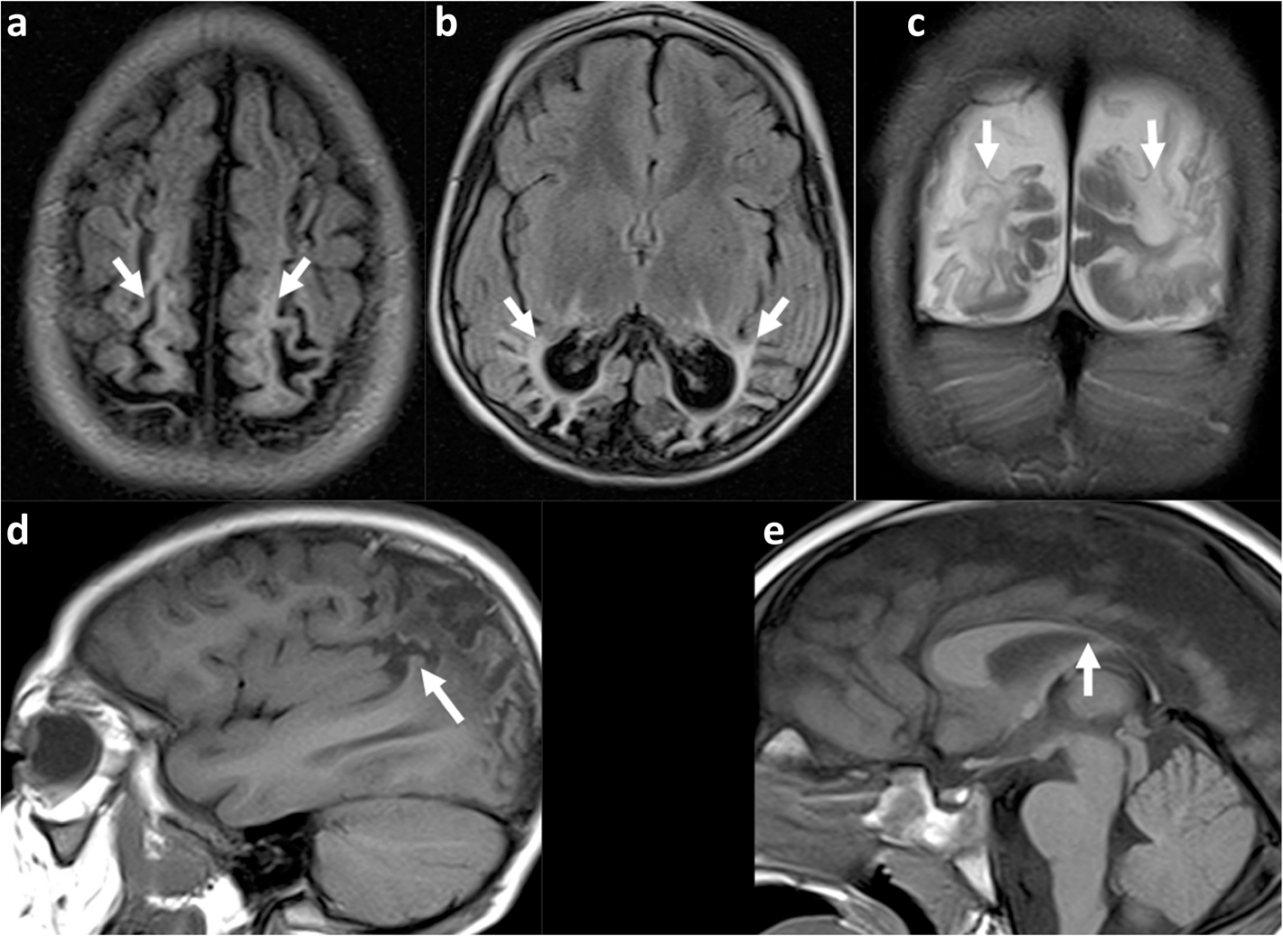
a–e Peri-Sylvian and posterior watershed injury in a 4-year-8-month boy. a, b Axial FLAIR images at two different levels which not only demonstrate the peri-Sylvian and posterior watershed distribution of the atrophy (arrows) but also demonstrate the underlying white matter signal abnormality up to the ventricular edge, with white matter volume loss. c Coronal T2-weighted imaging confirms the atrophy and signal abnormality of the posterior watershed in another plane (arrows) and demonstrates relative sparing of the occipital lobes just above the tentorium. d Sagittal T1-weighted image demonstrates the peri-Sylvian distribution of atrophy (arrow). e Sagittal midline T1 shows the marked volume loss of the posterior body, isthmus, and splenium of the corpus callosum (arrow) corresponding to cortical and white matter volume loss in the posterior watershed
